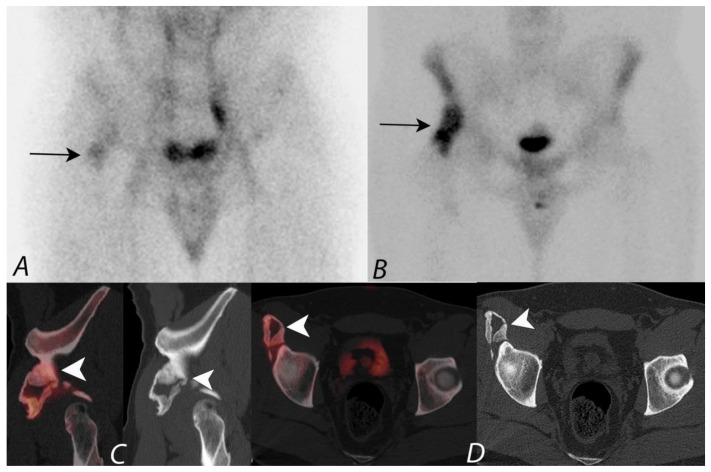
Patient presenting with right hip and with clinical history of possible heterotopic ossification. Planar blood pool image (A) and delayed image (B) in anterior projection from a ^99m^Tc MDP bone scan demonstrating increased blood pool activity and delayed focal uptake localizing to the right hip region (long black arrows). Fused sagittal image with correlative CT image (C) and fused coronal with correlative CT image (D) localizes the focal activity to an area of exuberant heterotopic ossification along the anterior aspect of the anterior wall of right acetabulum. Given increased blood pool activity, the heterotopic ossification is immature and represents an ongoing process.
